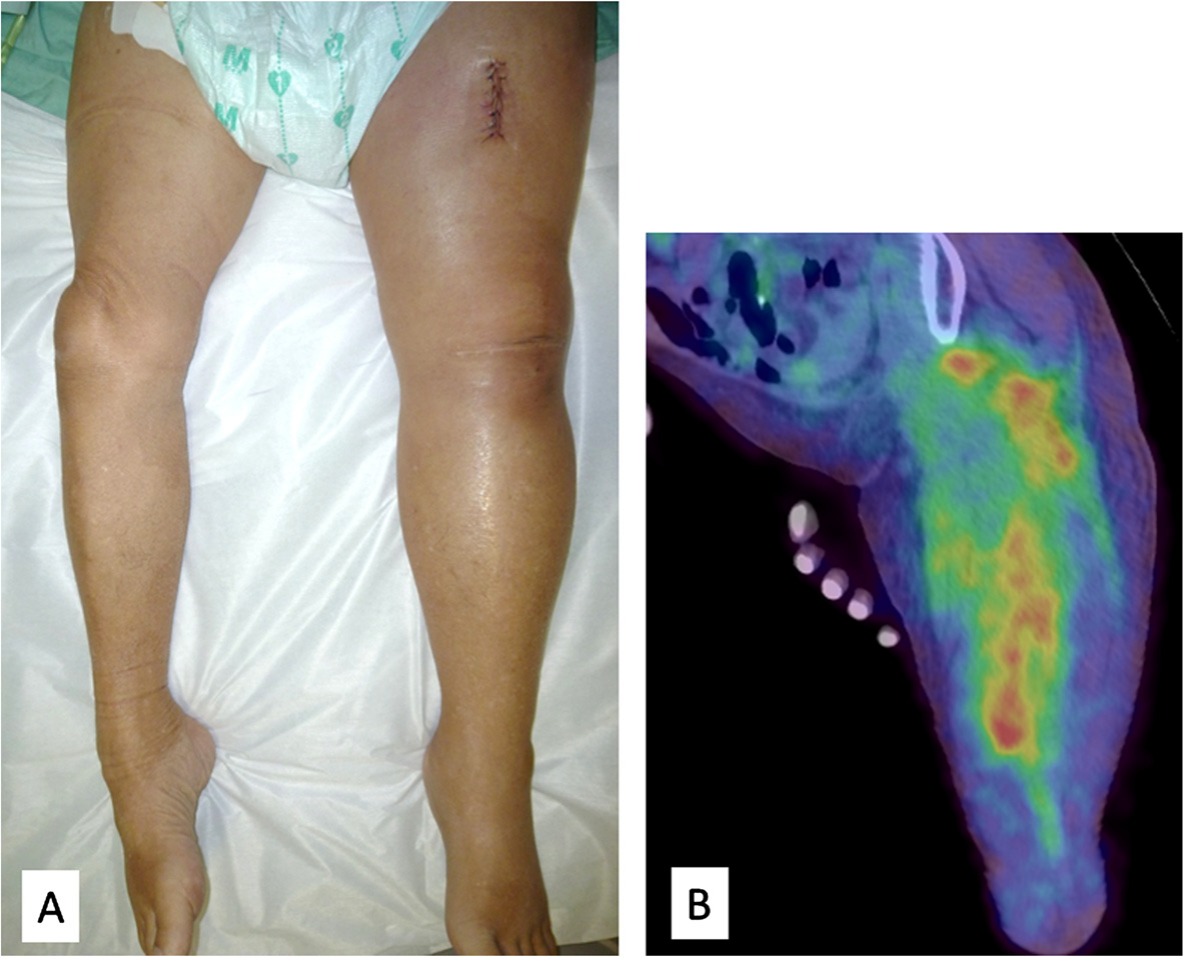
Photograph and positron emission tomography-computed tomography (PET-CT) image of the left femur. a Swelling of the left femur. An open muscle biopsy was performed. b PET-CT scan image showing increased metabolic activity in multiple skeletal muscles (latissimus dorsi, transverse, iliac, iliopsoas, and femoral)
